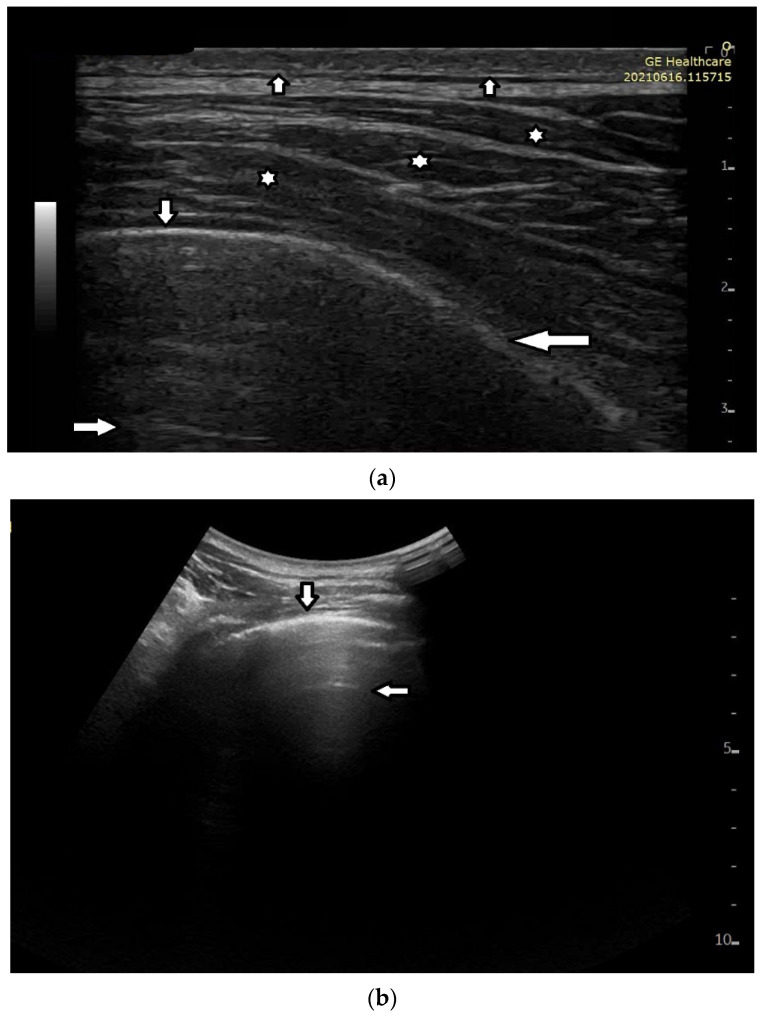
(a) Lung ultrasound, linear probe: white stars (*) muscles of the chest wall; down arrow (↓) well-defined pleural line (perpendicular ultrasound beam); left arrow (←) blurred pleural line secondary to the tangential incidence of the ultrasound beam; up arrow (↑) subcutaneous tissue; right arrow (→) A line, horizontal artifact. (b) Lung ultrasound, convex probe: down arrow—pleural line, smooth, echoic and regular; left arrow—A-line artifact.
